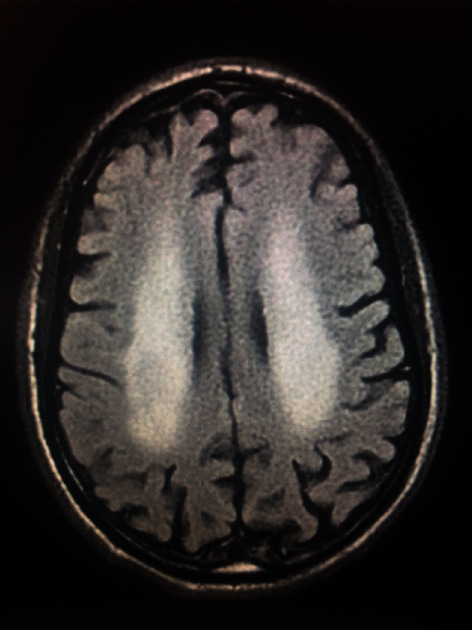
Brain MRI without contrast revealed symmetric hyperintense T2 FLAIR signaling in the periventricular white matter and the splenium of the corpus callosum.
